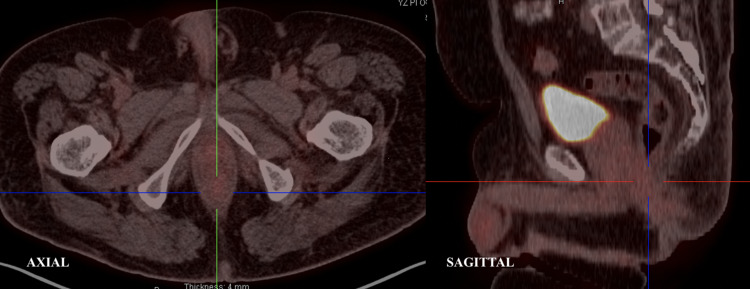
Axial and sagittal view of post-chemoradiotherapy PET-CT. PET-CT: positron emission tomography-computed tomography
